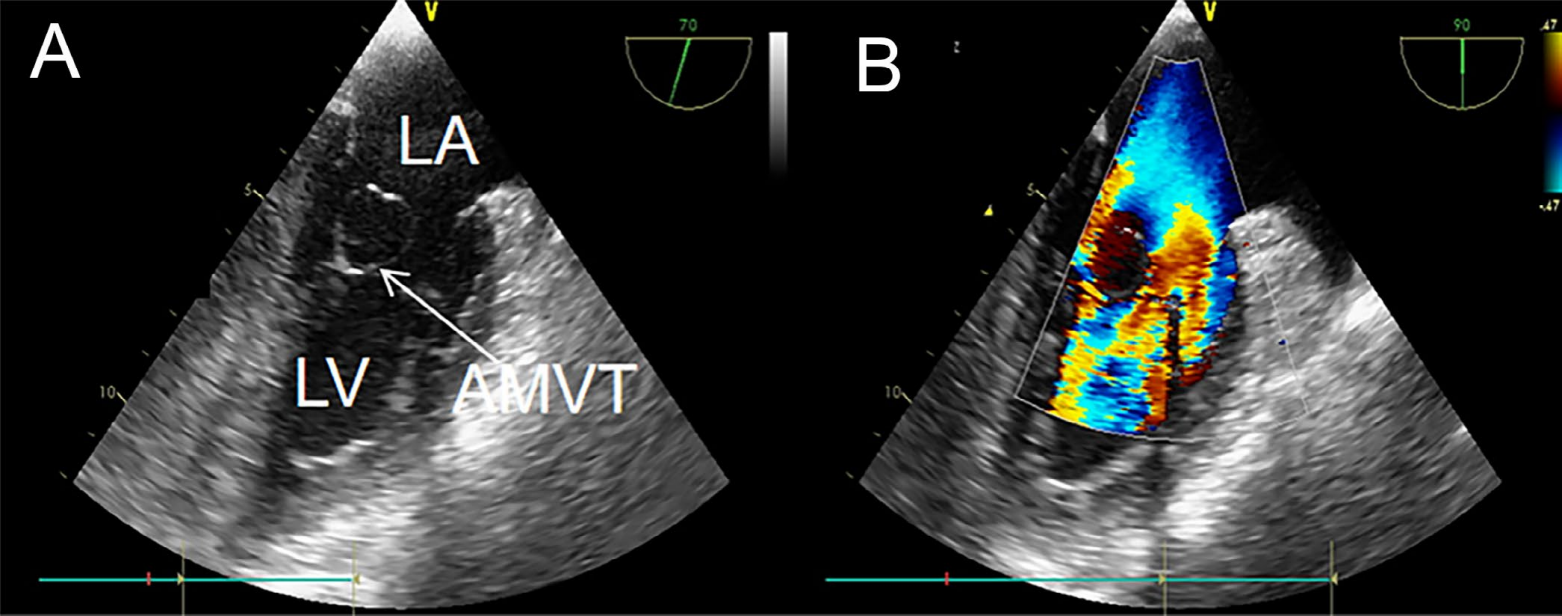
The features of case 1 on TEE and color Doppler. Systolic (A) phases of TEE can better display the anterior structure (shown by the white arrow in the figure). Color Doppler (B) shows no blood flow in the center of the mass and mild mitral regurgitation. LA, left atrium; LV, left ventricle.
